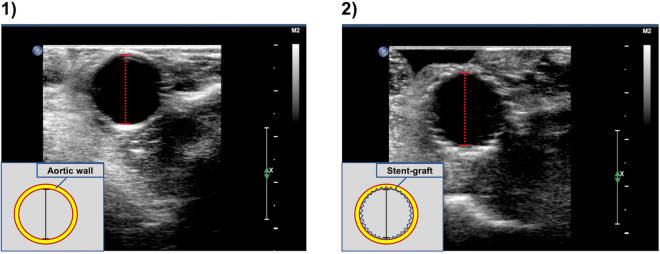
Ultrasound imaging of a cross section of the aorta at baseline (1) and after EVAR (2). The internal aortic diameters at the stent-graft site were measured as aortic diameter at baseline and after EVAR.
